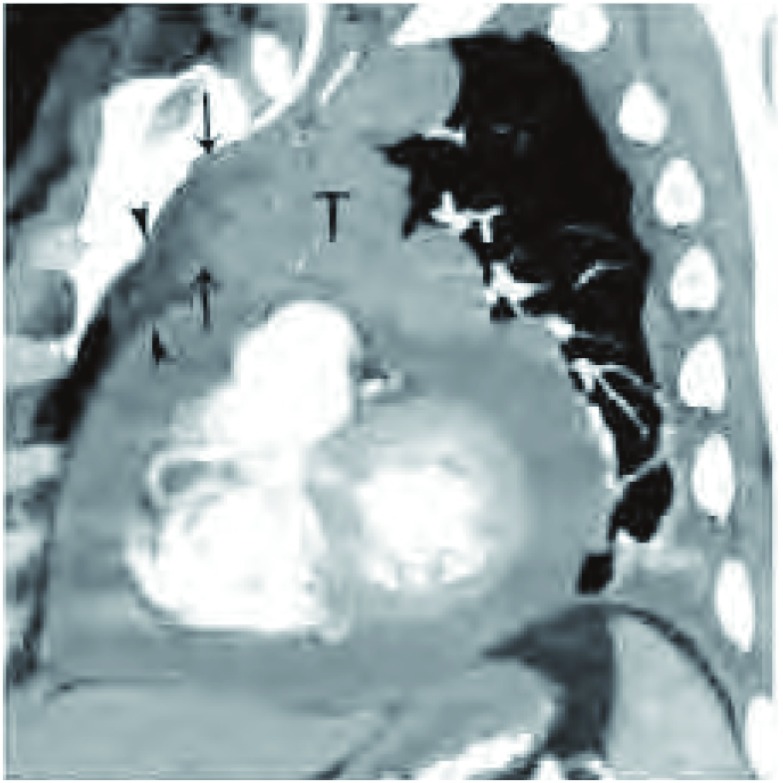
侵犯纵隔脂肪。增强CT冠状位重建（软组织窗）所示，胸腺瘤（T）侵犯纵隔脂肪组织（黑箭头所示）。注意，远离肿瘤的纵隔脂肪密度低于紧邻肿瘤的脂肪密度。 Infiltration of mediastinal fat. Coronal oblique reformatted contrast-enhanced chest computed tomography (CT) (soft tissue window) shows the thymoma (T) with infiltration of surrounding mediastinal fat (black arrows). Notice that the attenuation of the mediastinal fat further away from the tumor (arrowheads) is lower than that immediately adjacent to the lesion.
